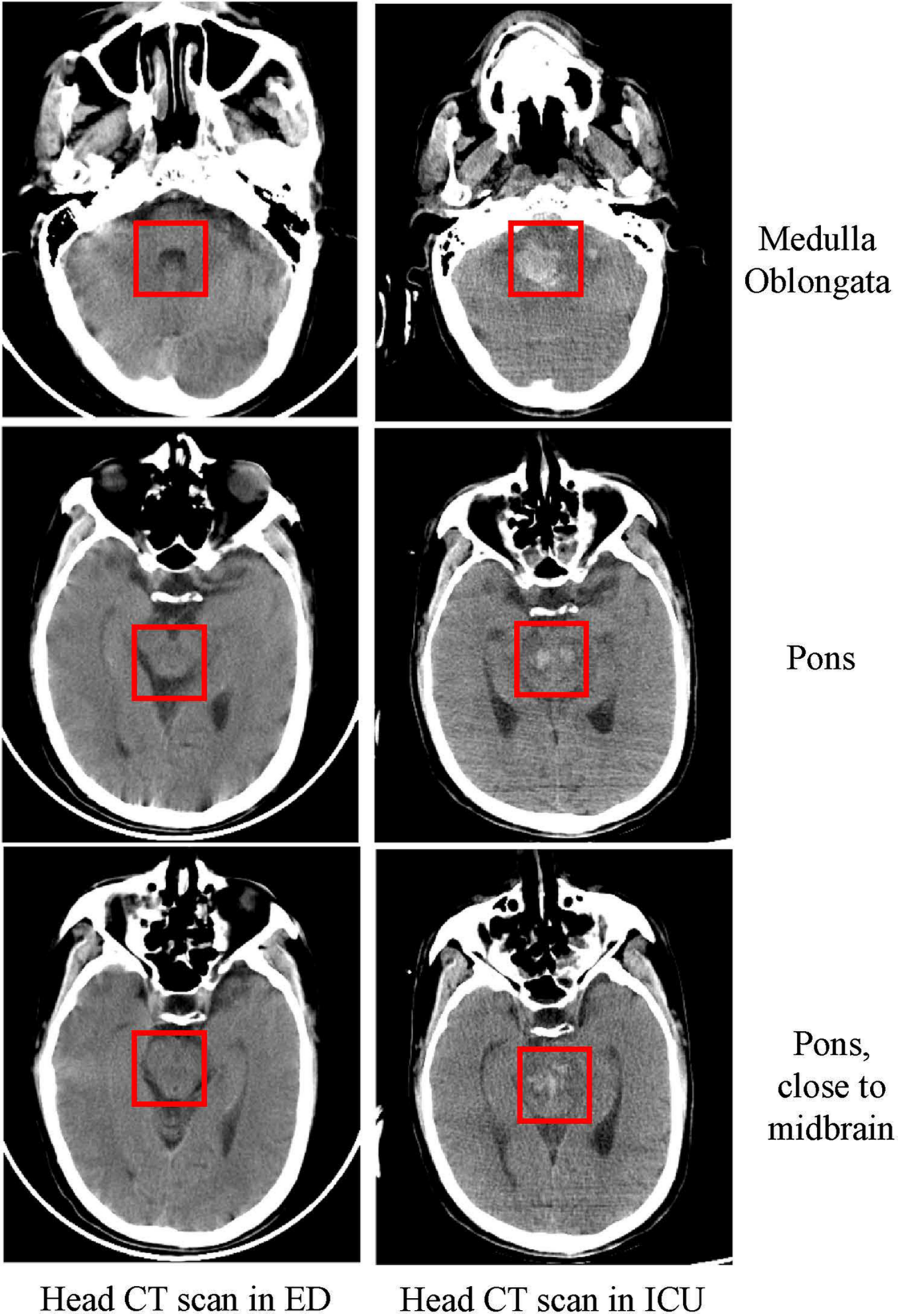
Head CT scan in the ED and ICU. Head CT scan showing that as the disease progressed within five days, the patient had extensive bleeding from the medulla oblongata to the pons as marked in the figure.
